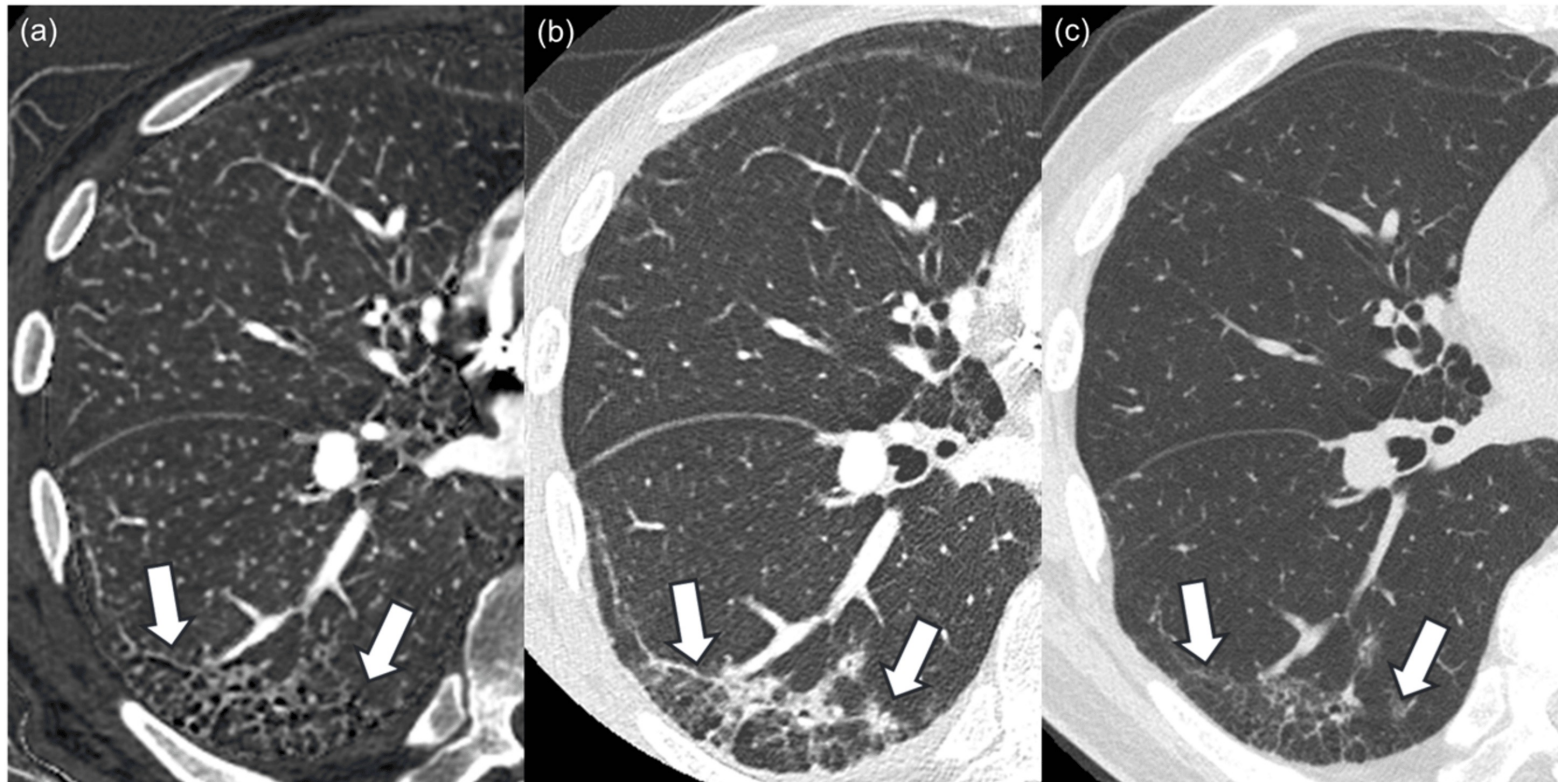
Images of a patient diagnosed with anti-melanoma differentiation-associated gene 5 antibody-positive dermatomyositis. The iodine map (a) and monochromatic image (b) of a 55-year-old female patient diagnosed with anti-melanoma differentiation-associated gene 5 antibody-positive dermatomyositis. Bronchiectasis and airspace enlargement, which are presented as black tiny structures in the region surrounded by white arrows, are more prominent in the iodine map compared to the monochromatic image, and readers 1, 2, and 3 rated 2 (iodine map is strongly prominent) in side-by-side qualitative evaluation. Bronchiectasis and airspace enlargement were ameliorated in the evaluated region in the follow-up CT examination (c), which was performed after 468 days. Window level/width for iodine map (a) and CT images (b, c) were 15/110 mgI/ml and –600/1600 Hounsfield unit, respectively.
